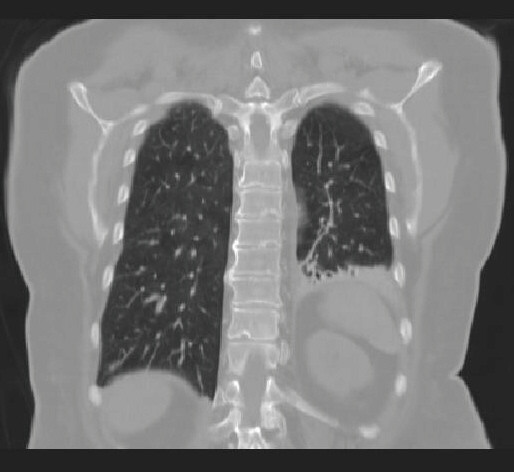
Coronal section of the chest (CT-scan) showing multiple lung metastases.
